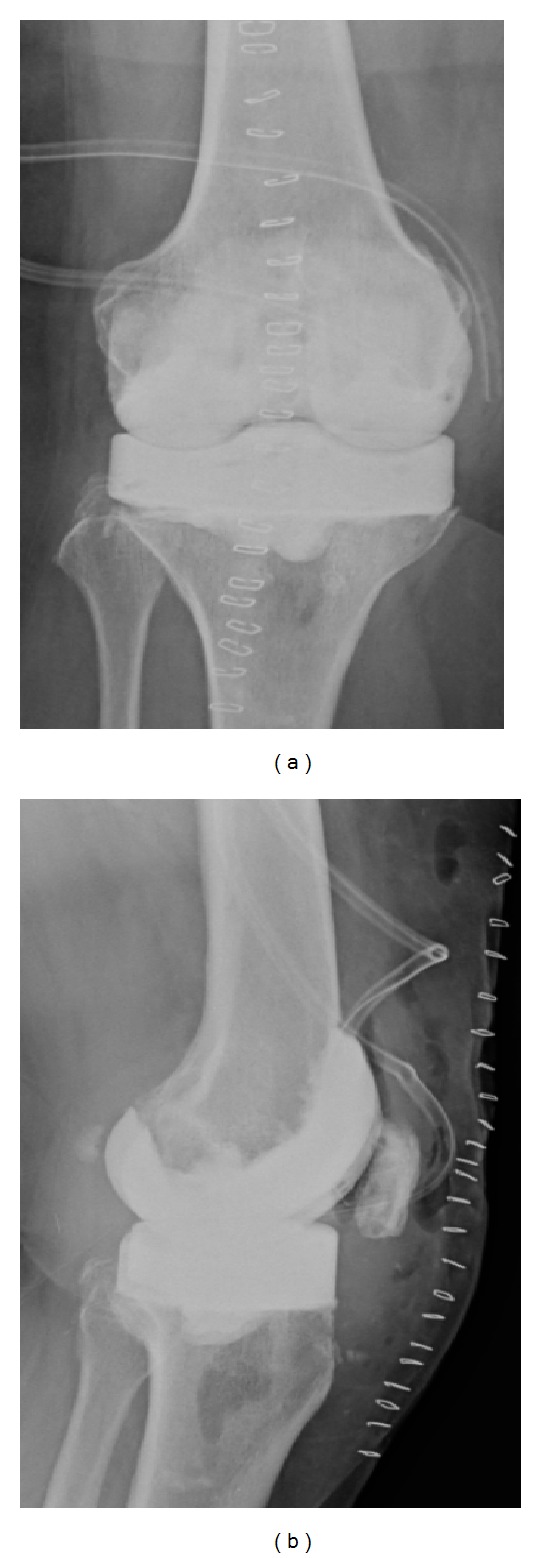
X-ray images showing* (a) anterior posterior view with the CLPS implanted and (b) lateral view with the CLPS implanted.
